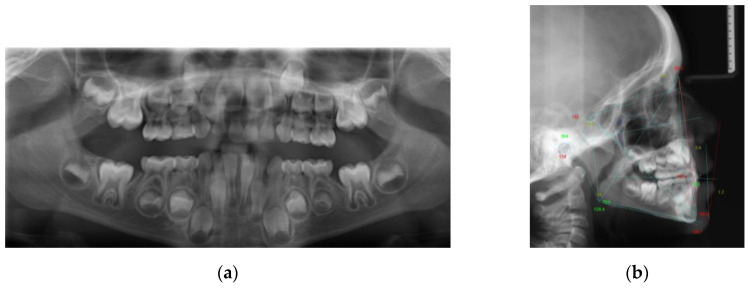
Pretreatment radiographic records: (a) orthopantomogram; (b) lateral cephalogram.
